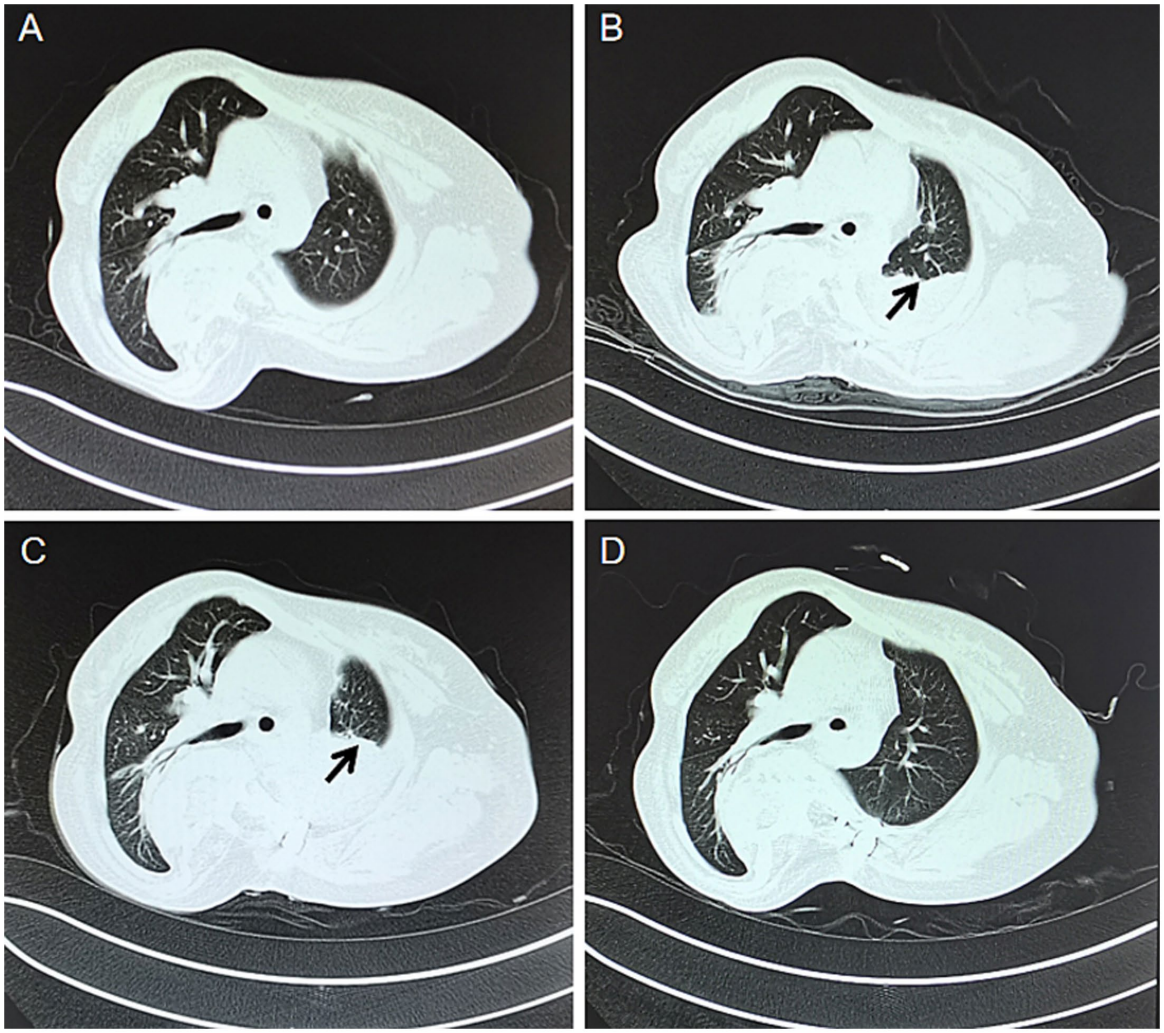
CT images of the patient’s chest at different times. (A) Preoperative. (B) Post-surgery at 2 days. (C) Post-surgery at 13 days. (D) One week after airway clearance treatment. The black arrow points to the location of pulmonary atelectasis in the patient’s left lung.
